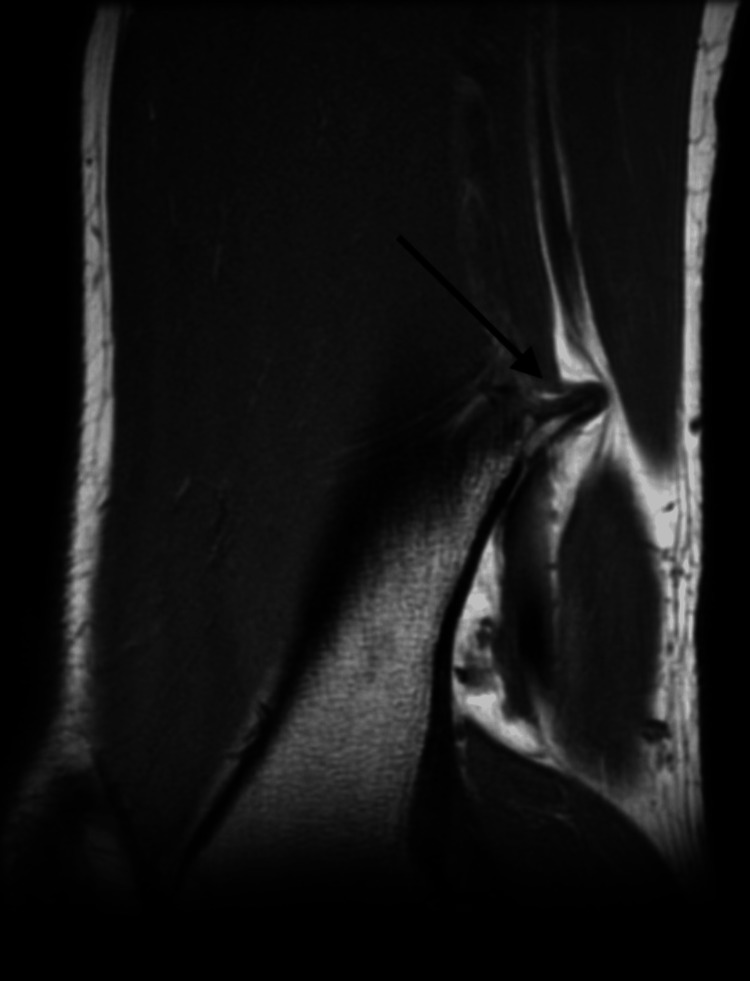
MRI T1-weighted sagittal imaging showing osteochondromas and their relation to the femur and neurovascular structures
